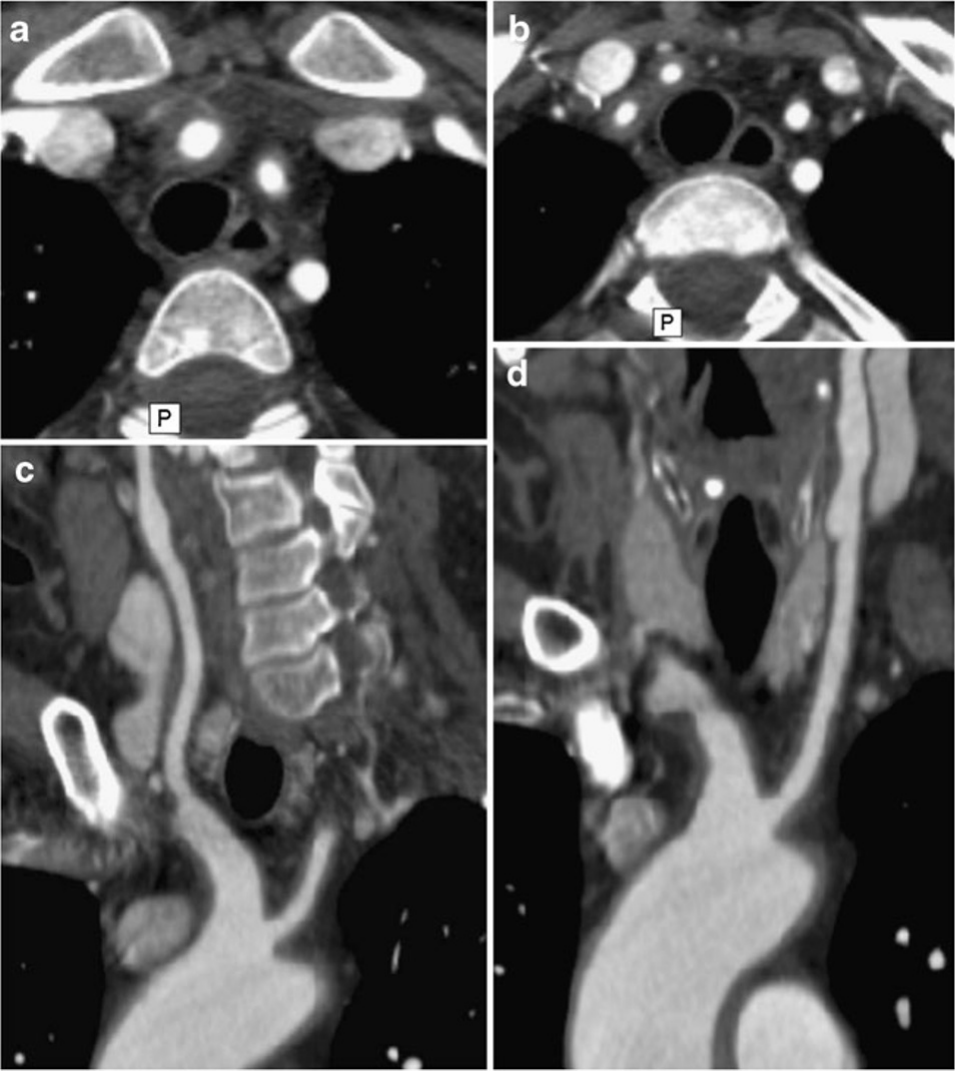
a–d A 48-year-old woman with weight loss, malaise and headaches. Axial (a, b) CTA images demonstrate concentric thickening of the brachiocephalic artery, continuing toward its branches: right common carotid artery, right subclavian artery and left subclavian artery (originating from the brachiocephalic artery in this case). Multiplanar curved reformats (c, d) demonstrate both wall thickening and aneurysmal dilatations along the course of both common carotid arteries (c*right*, d*left*) and brachiocephalic artery trunk (c). Courtesy of Dr. Ludmila Guralnik, Rambam Health Care Campus, Haifa, Israel
